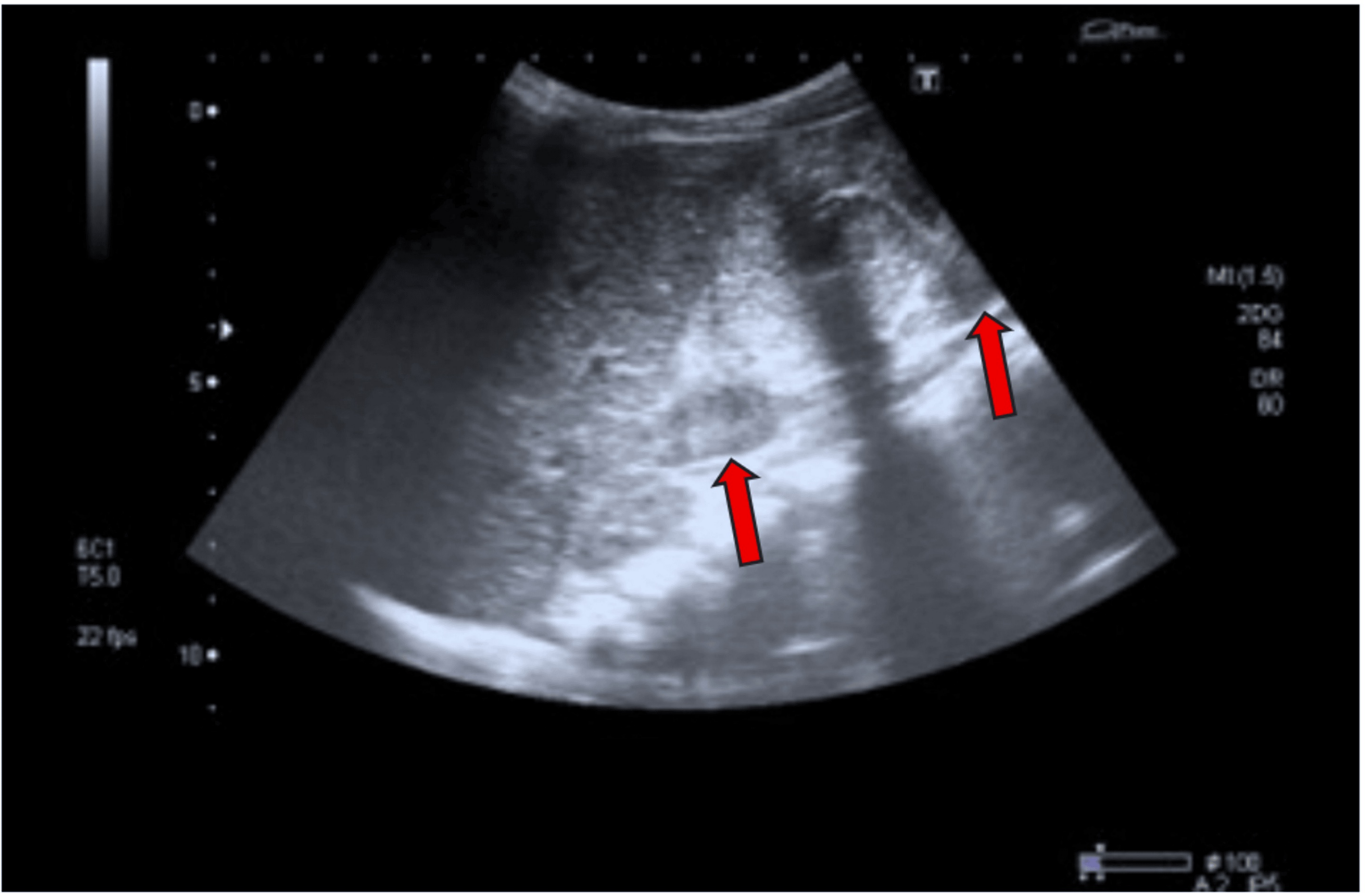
Ultrasound image showing bilateral mesenteric lymph nodes (LNs), measuring 13 × 6 mm on the right side and 17.4 × 4.7 mm on the left side (red arrows).
